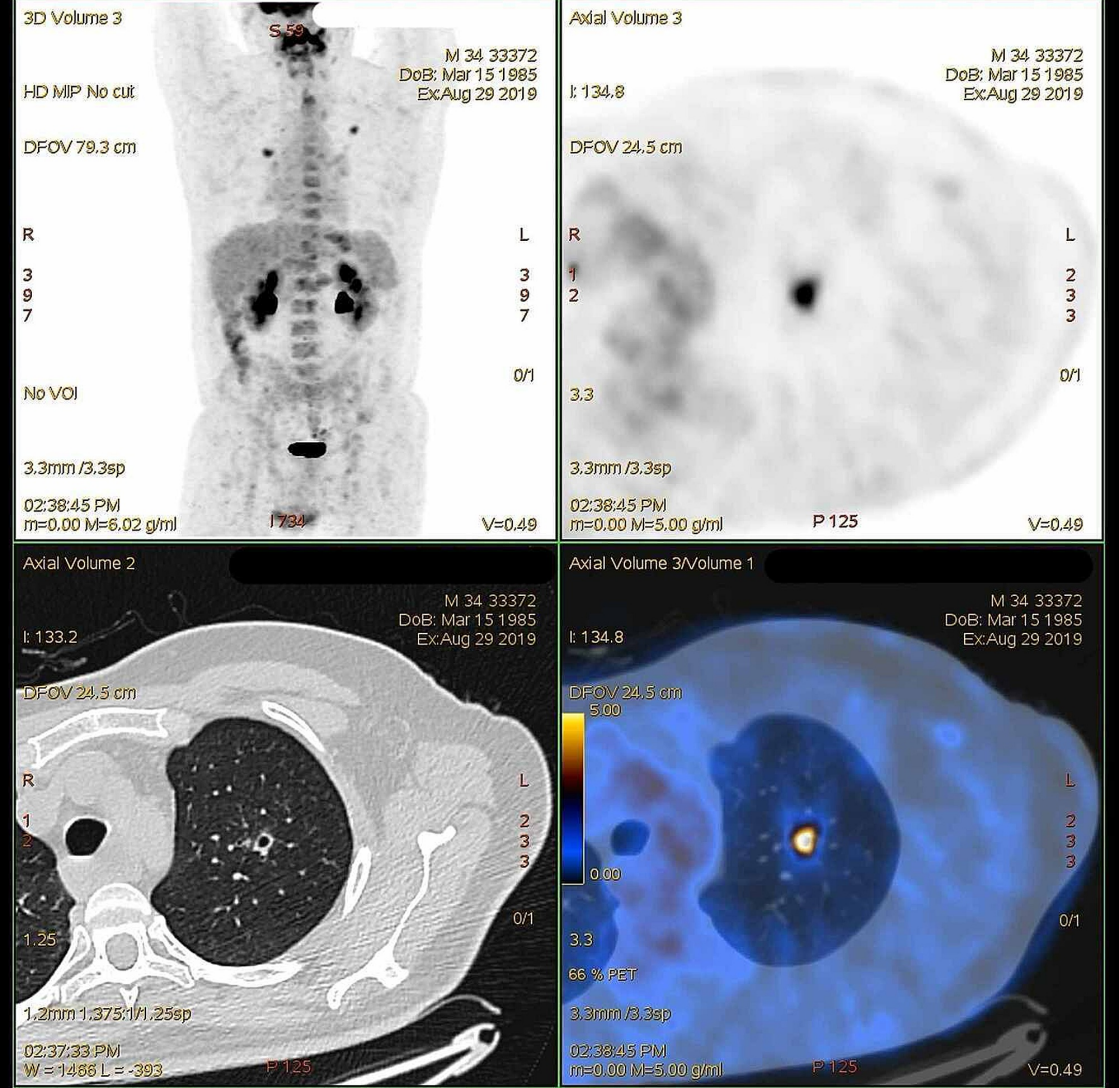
PET-CT Identification of a hypermetabolic nodule in the upper left lobe, with irregular contours, cavitated, measuring about 16 mm. There are also several nodules in the left lung, with limited functional characterization due to their small dimensions. PET-CT: positron emission tomography-computed tomography
